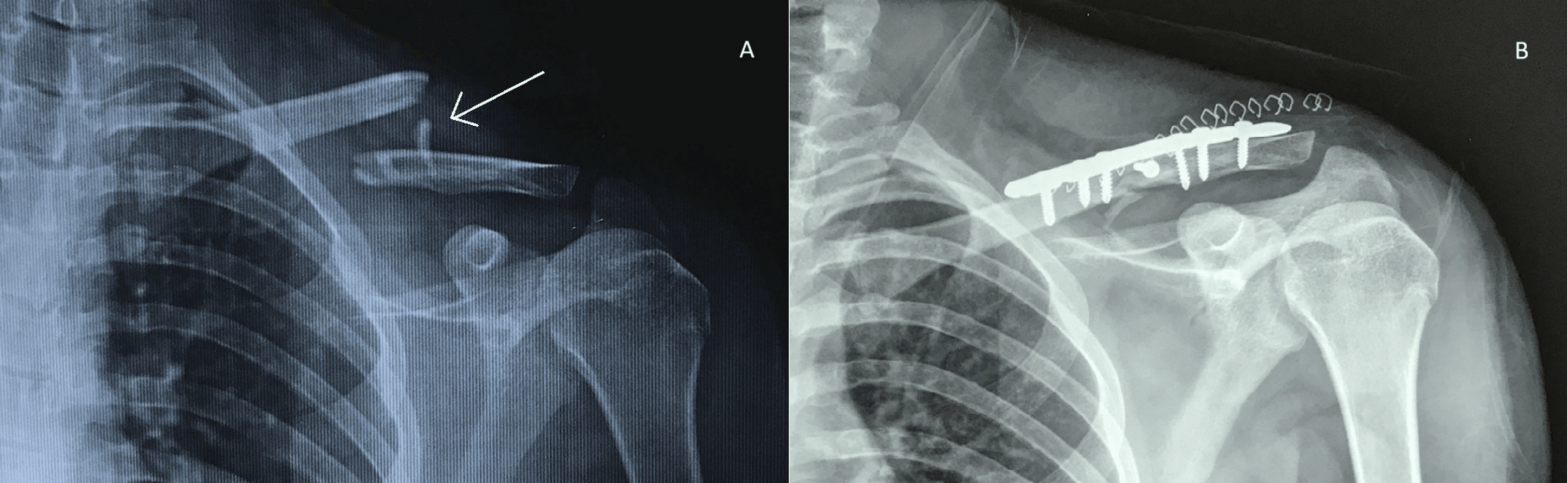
Pre- and post-operative x-rays of patient 17. (A) Pre-operative image with the often-seen anterosuperior butterfly fragment (arrow). (B) Post-operative image showing fixation construct.
